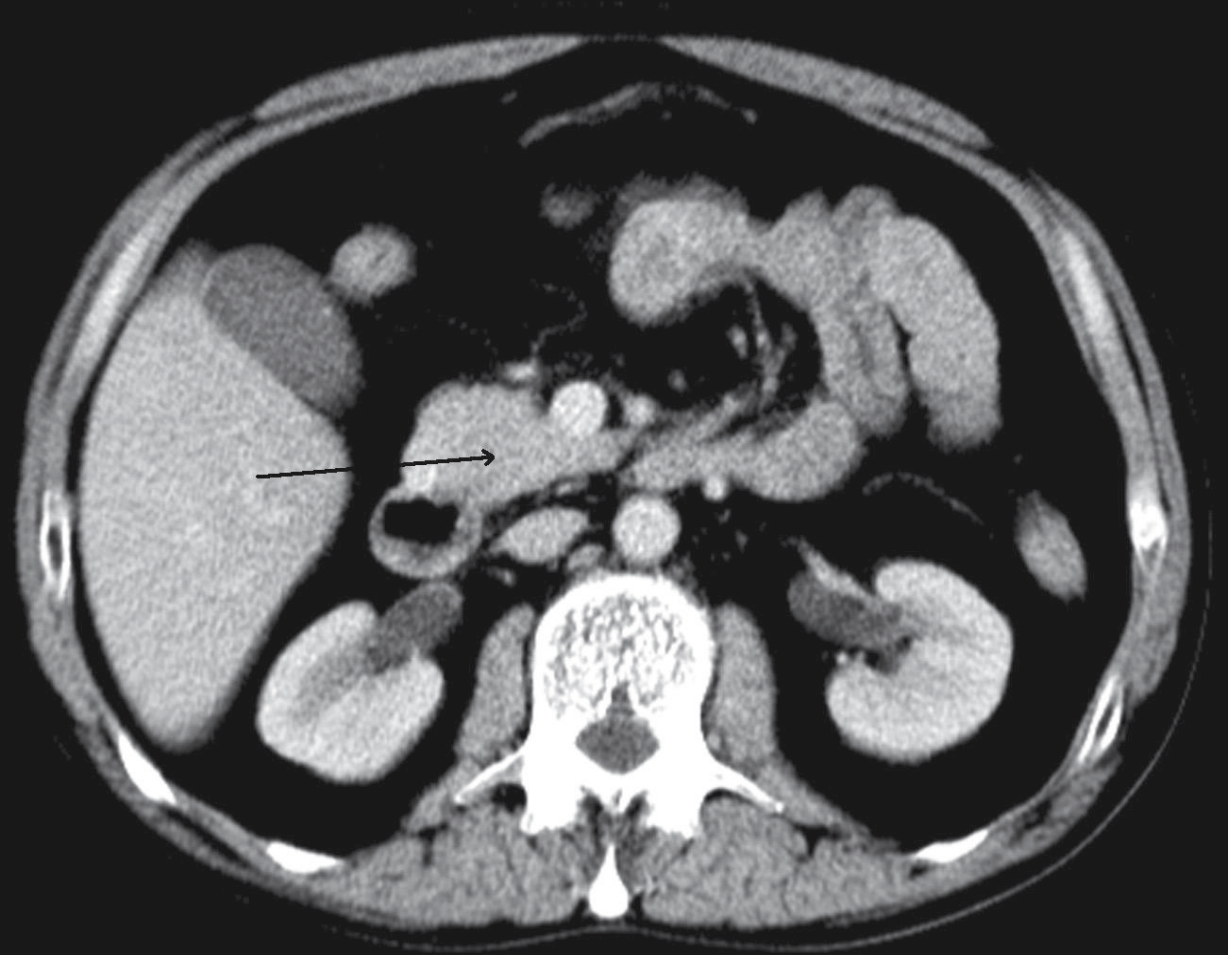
Axial contrast-enhanced CT image shows a normalappearing truncated head with a normal-appearing uncinate process (black arrow)
